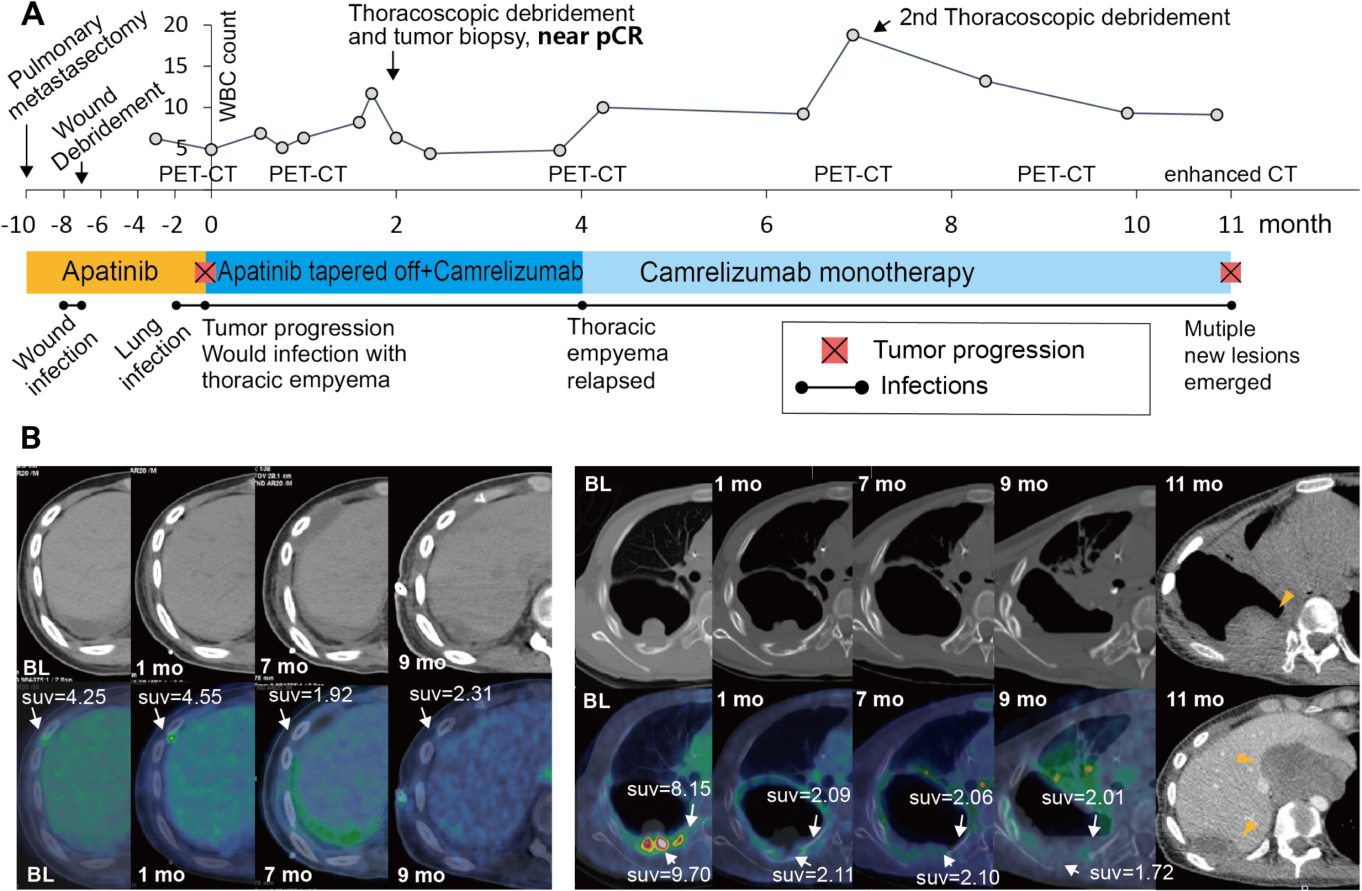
The timeline of the patient’s infection, immunotherapy and oncological outcomes. (A) The patient has previously developed wound infection and lung infection, and had ongoing wound infection with thoracic empyema when initiating the PD-1 inhibitor Camrelizumab. The change of the serum white blood cell (WBC) count and 3 surgical debridement procedures during the course of the immunotherapy are denoted. Tumor biopsy during the thoracoscopic debridement (2 months post-immunotherapy) suggested a pathological near-complete response of the tumor. (B) The FDG PET/CT images of the metastatic lesions before and during the course of the immunotherapy. The pleural and costal metastases demonstrated a rapid and durable response to Camrelizumab (white arrow). Enhanced CT scans at 11 months post-treatment suggested pleural tumor relapse (yellow arrowhead) as well as multiple new lesions in the mediastinal (yellow arrowhead), pleural (yellow arrowhead) and inferior vena cava (not shown) regions.
